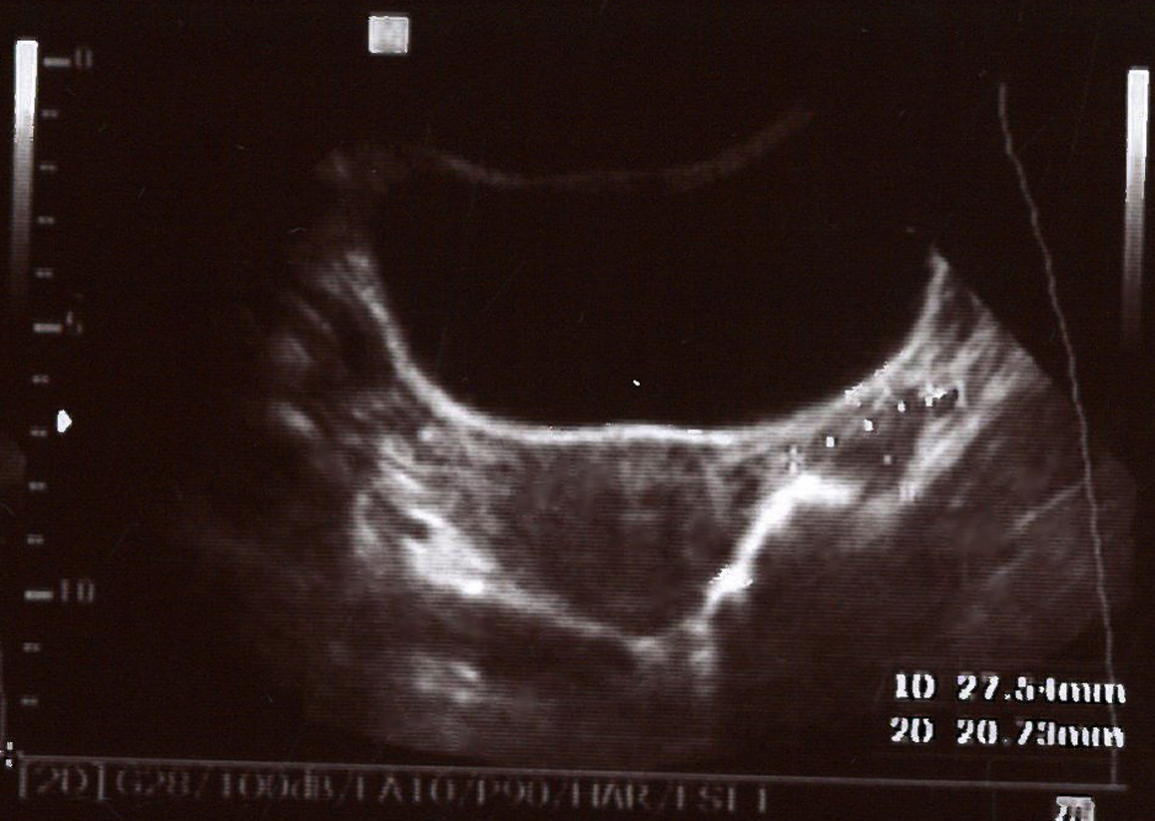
In the sonography, a 16 × 32 polypoid lesion is seen in the posterior wall of the bladder (in the center of the Figure).
